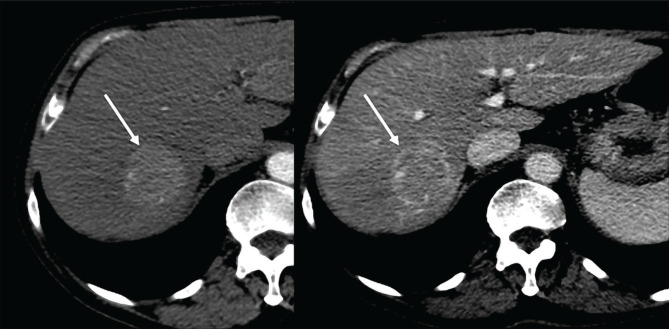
Enhanced CT, arterial and portal phase. A large hepatic nodule (5 cm) is seen in the VIIth segment, with arterial enhancement and rapid washout in portal phase (arrow), a typical CT appearance of HCC.
